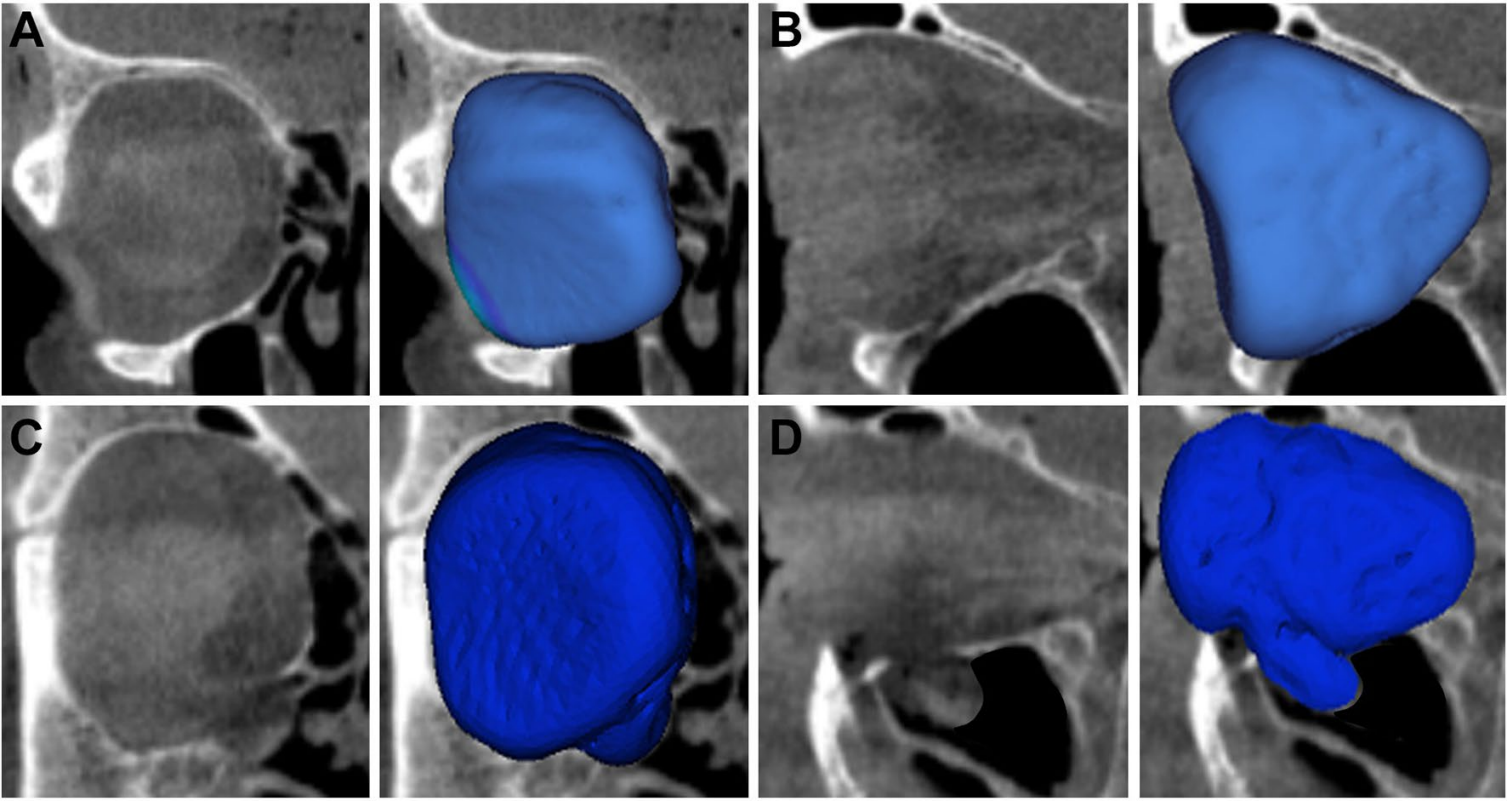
Intact and fractured orbits. Cone-beam CT scans of the right orbit in coronal (a, c) and sagittal (b, d) view. Segmented volumes of intact (light blue) and fractured orbits (dark blue) were automatically created by specialized software
